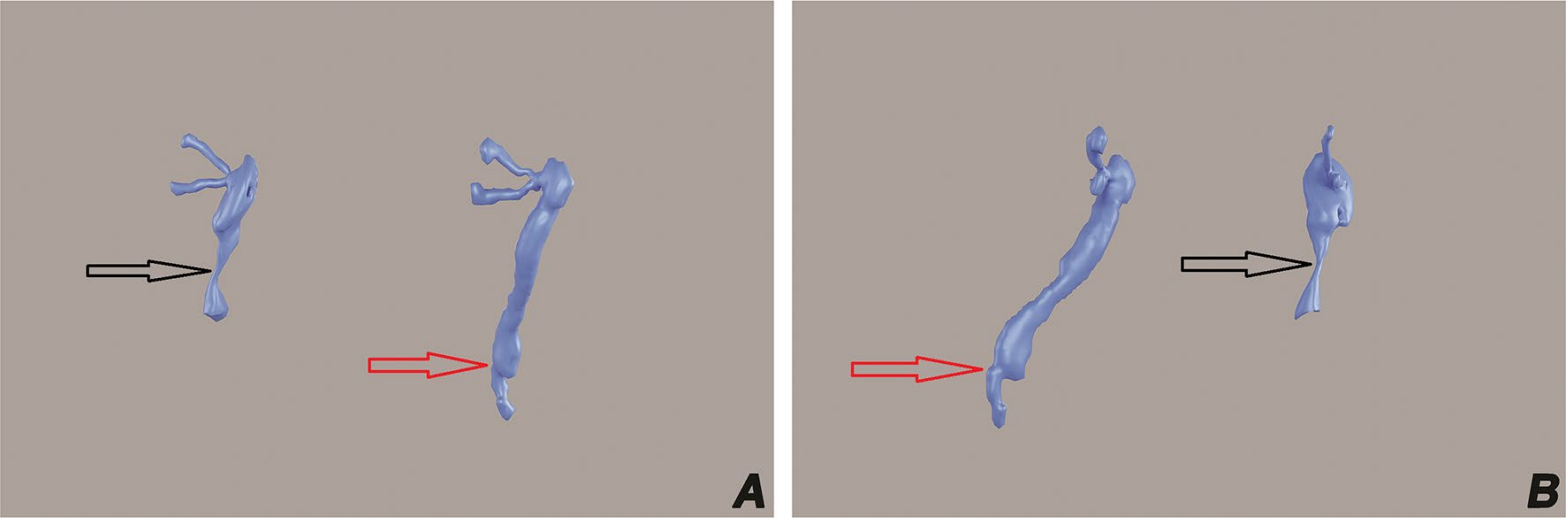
CT-DCG reconstruction: Three-dimensional reconstructions of a CT-DCG showing anterior view (Panel A) and lateral view (Panel B). Note two lacrimal sacs, one of them in each panel shows a gross narrowing at the middle of the nasolacrimal duct (black arrow, Panels A and B). This narrowing is smooth without change of angulation and would be easier amenable to BDCP. The other lacrimal sac shows abrupt change of angulation following the stenosis (red arrow, panels A and B) and proximal dilatation. These cases would be difficult technically during a BDCP procedure.
